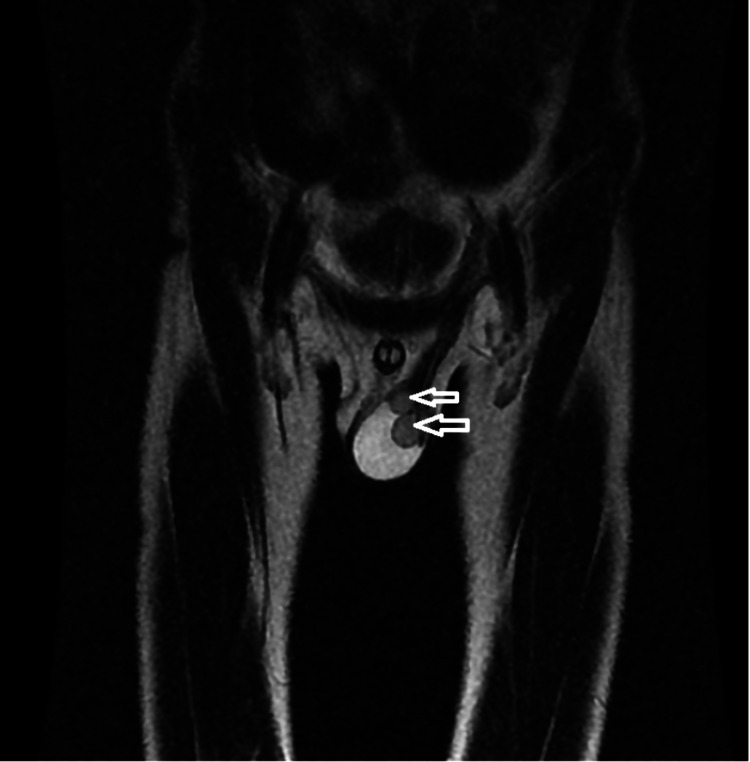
T2WI MRI image coronal section showing moderate left-sided hydrocele with left testis and another testis at the root of the scrotum on the left side (white arrows). T2WI: T2-weighted image.
